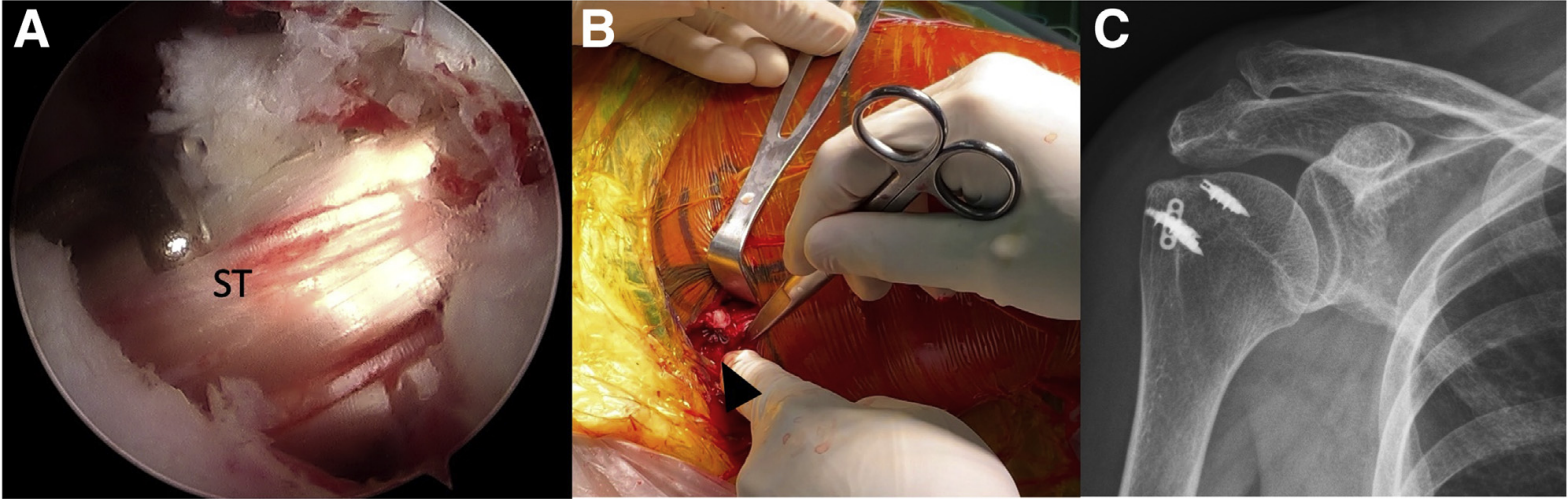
Tensioning of lower trapezius tendon and semitendinosus graft, right shoulder. (A) After the EndoButton is flipped and fixed at the bicipital groove, the tension of the ST graft is checked intra-articularly under arthroscopy. (B) The other limb of ST graft is fixed side by side with the LTT with Krakow suture (arrow). (C) Postoperative x-ray revealing EndoButton fixed inside bicipital groove. Abbreviations: LTT, lower trapezius tendon; ST, semitendinosus.
